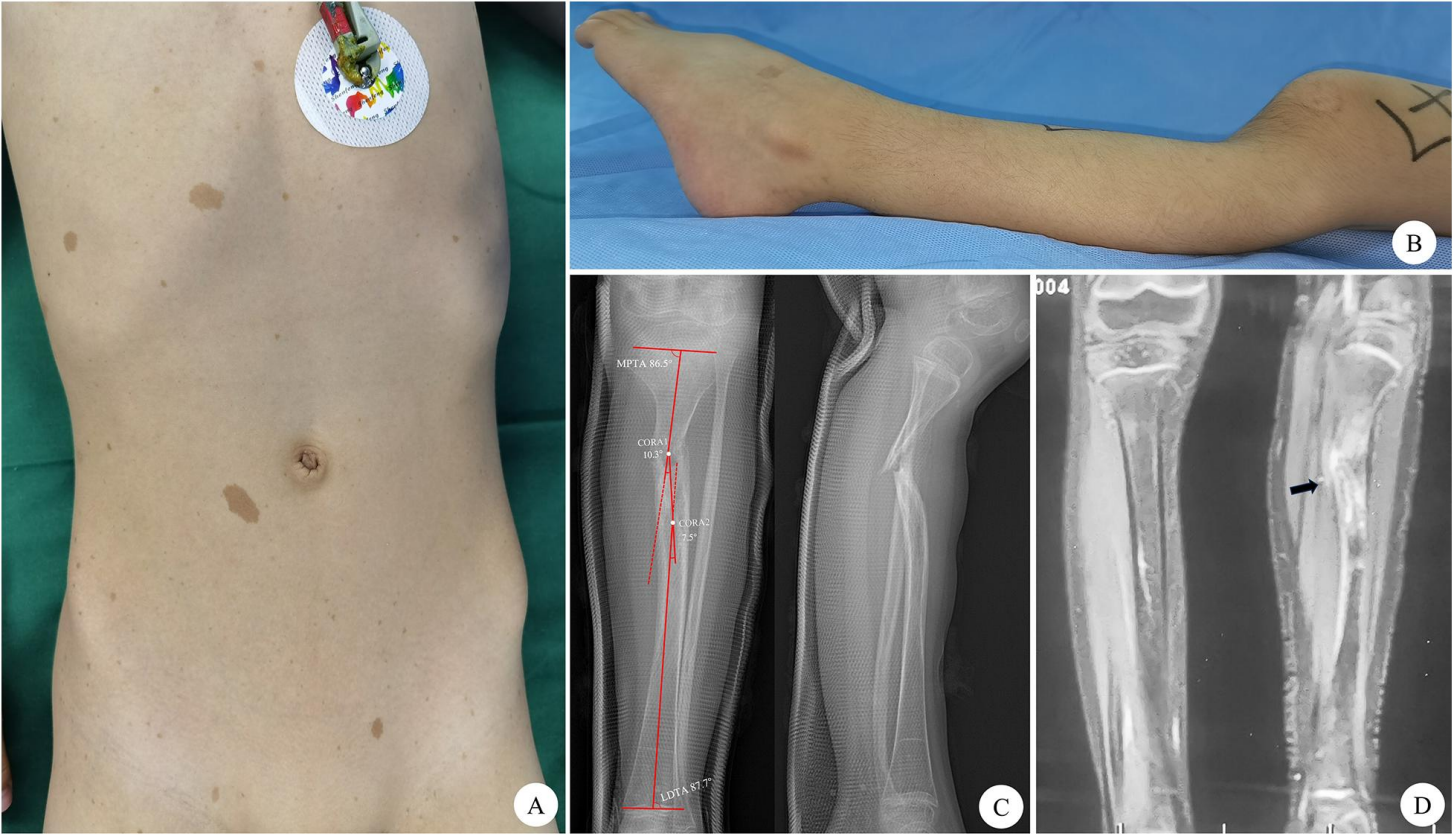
Preoperative physical examination and imaging findings of the child. (A) Café-au-lait macules visible on the chest and abdomen. (B) Posterior angulation deformity of the proximal left lower leg. (C) Preoperative anteroposterior and lateral radiographs demonstrate a pseudarthrosis in the proximal third of the tibia, associated with characteristic proximal tibial dysplasia, manifesting as trumpet-shaped narrowing. Bone atrophy and sclerosis at the bone ends of the pseudarthrosis are also evident. (D) T2-weighted MRI of both lower limbs, with black arrows highlighting abnormally high signal intensity in portions of the tissue surrounding the pseudarthrosis.
